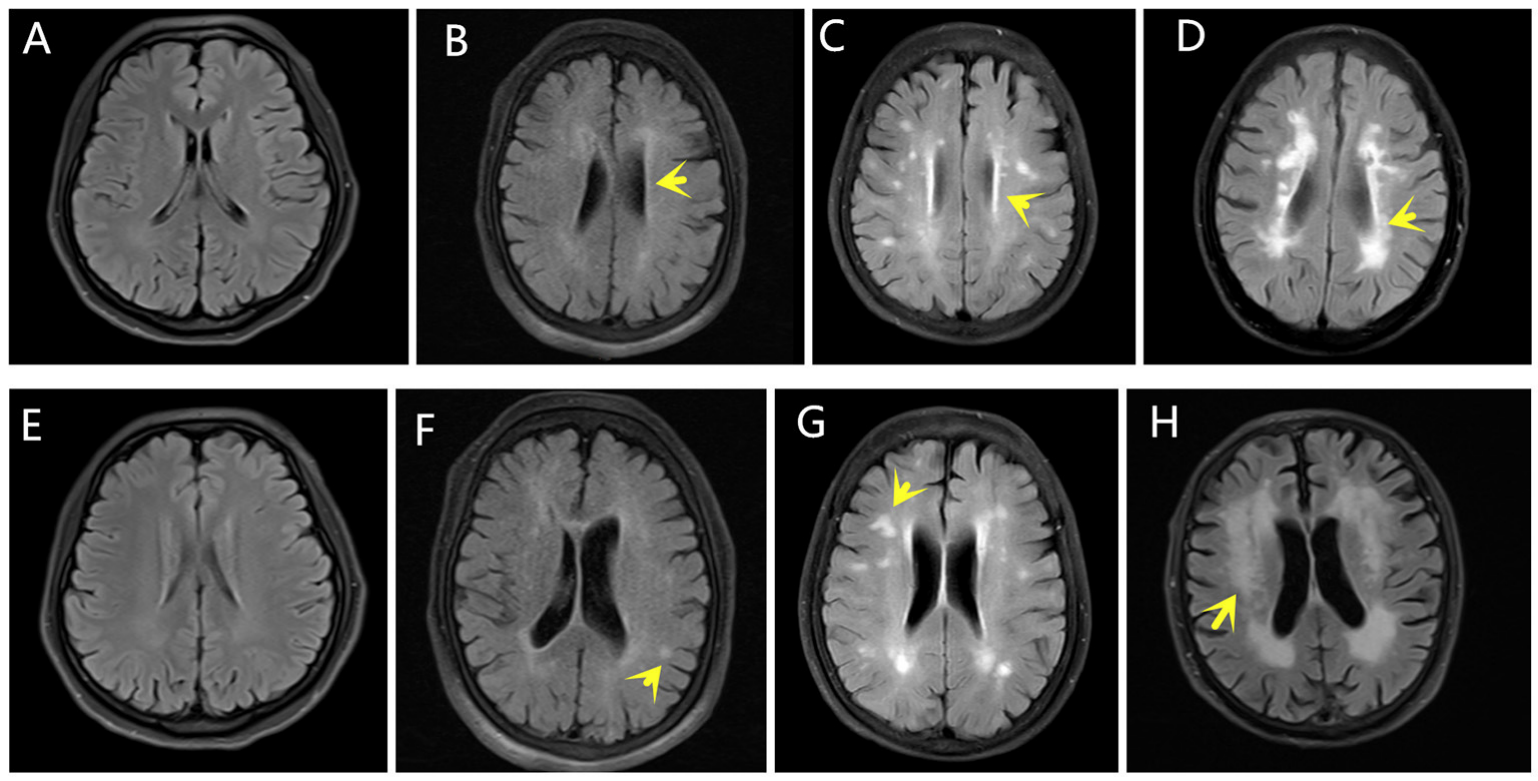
Fazekas scores. The yellow arrows indicate WMLs on FLAIR MRI. The PV scores were categorized as follows: (A) 0, (B) 1, (C) 2, (D) 3. The DWM scores were categorized as follows: (E) 0, (F) 1, (G) 2, (H) 3.
